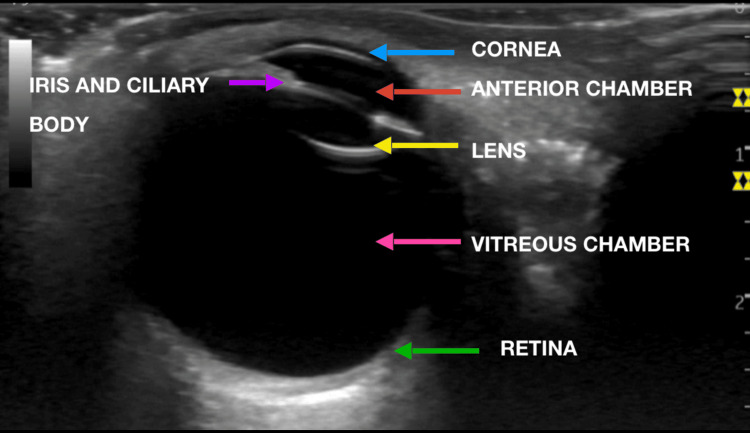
Ocular POCUS of the normal right eye. POCUS: point-of-care ultrasound
